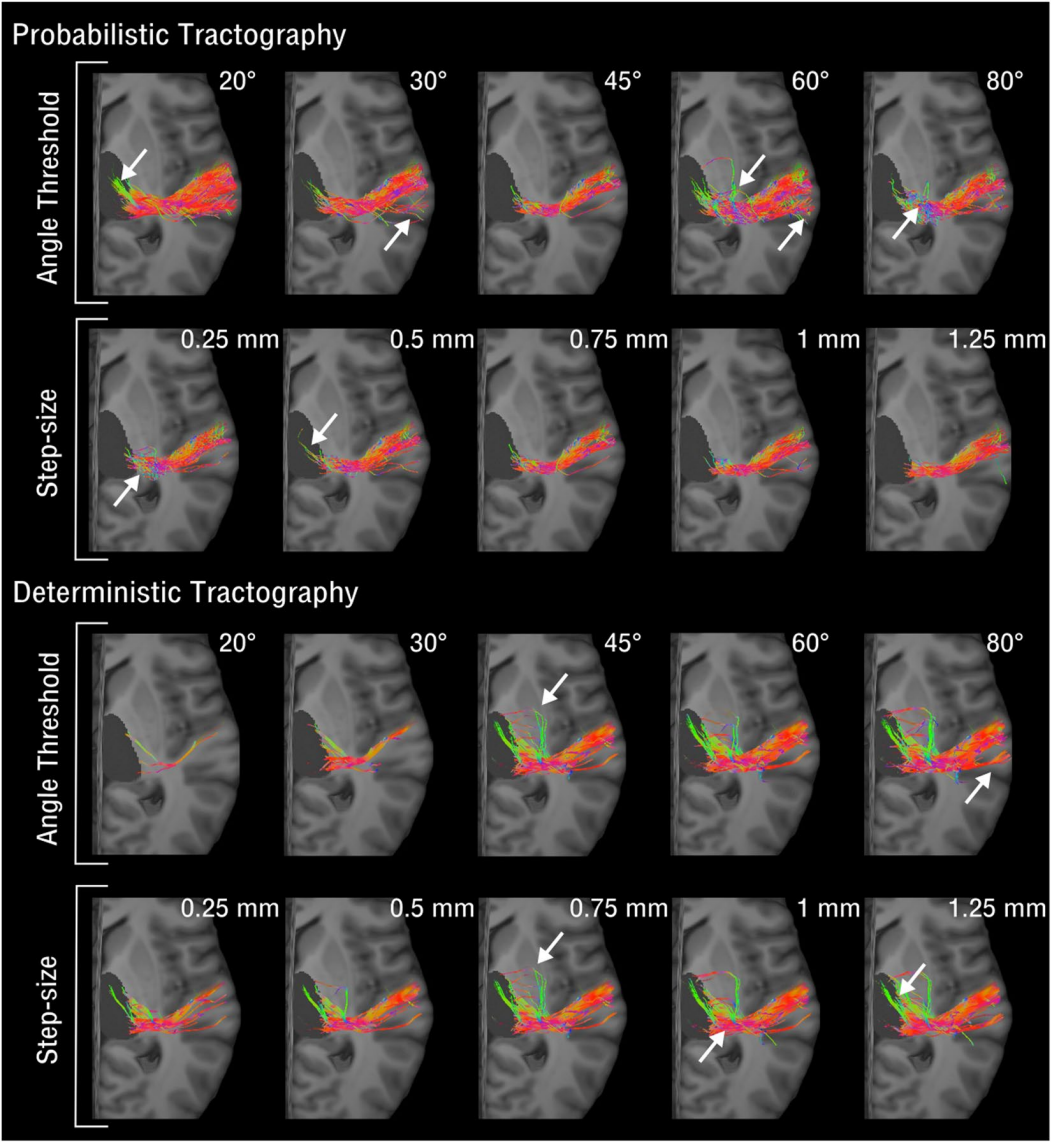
Tractography Parameters Effects on the Acoustic Radiation Reconstruction. The image shows the effects of tractography parameters (angle threshold and step size) on the reconstruction of the right hemisphere acoustic radiation for both probabilistic and deterministic algorithms on a representative subject using an MRI diffusion acquisition with b = 10,000 *s*/*mm*^2^. White arrows point to false positives in the tractography reconstructions. The reconstructed streamlines are shown overlaid on the T1-weighted image of the subject. The streamline colour encodes directionality (anterior posterior: green, superior-inferior: blue, left-right: red). The 3D rendering of the right thalamus is also shown in dark grey.
